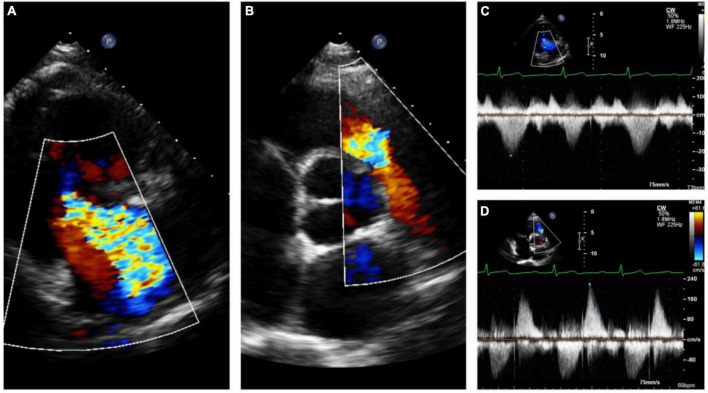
Echocardiography monitoring in carcinoid heart disease (CnHD): Pre-surgical baseline echo. Severe tricuspid regurgitation (A) with pulmonary valve stenosis (B) with corresponding continuous wave Doppler (C, D).
